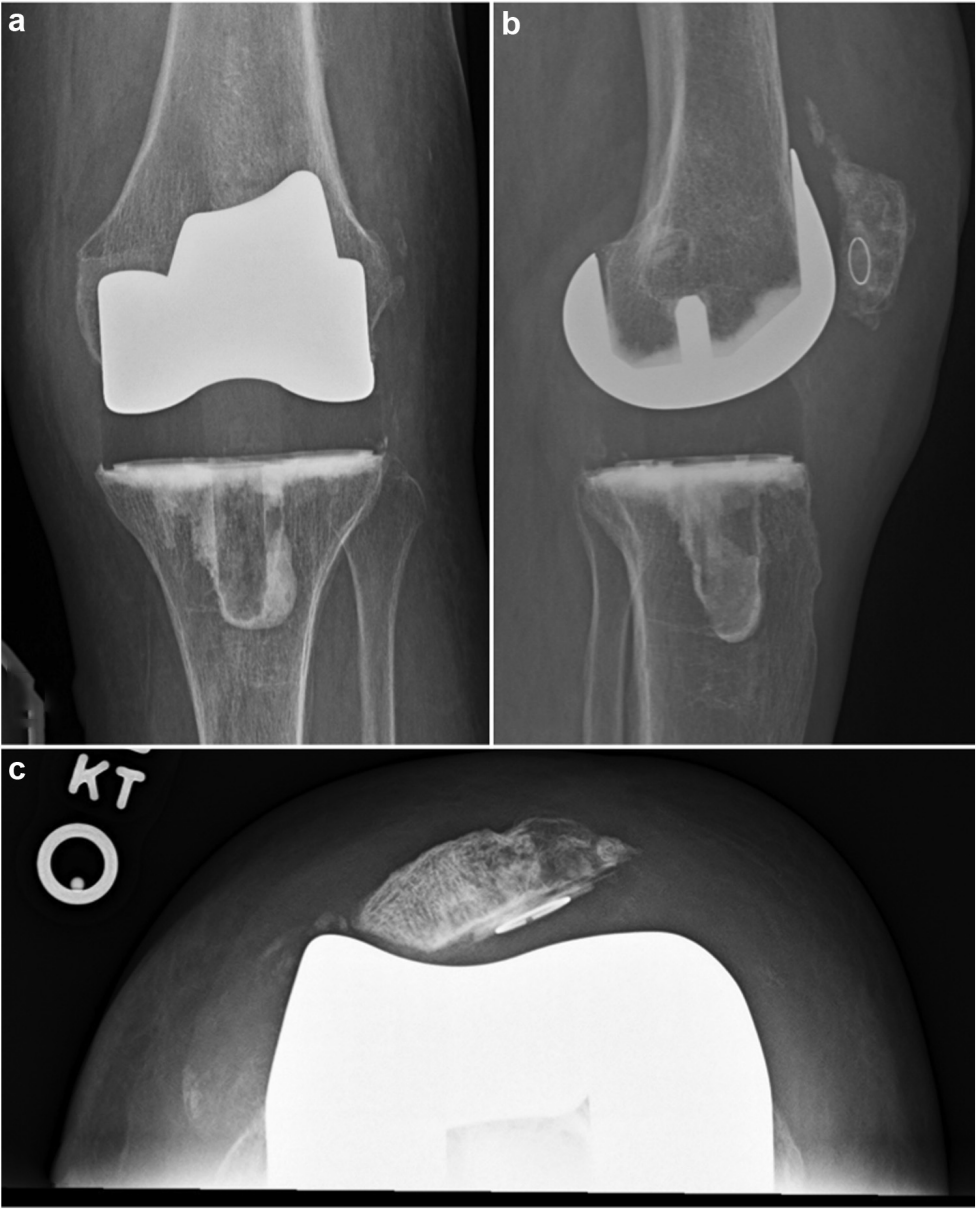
Six-months follow-up X-rays. Anteroposterior (a), lateral (b), and merchant view (c). She has active range of motion from 0° to 115° of flexion. She does have notable lateral patellar tilt in her trochlear groove, but the patella remains centered over the trochlea despite the excessive lateral tilt. No evidence of subluxation or frank dislocation.
